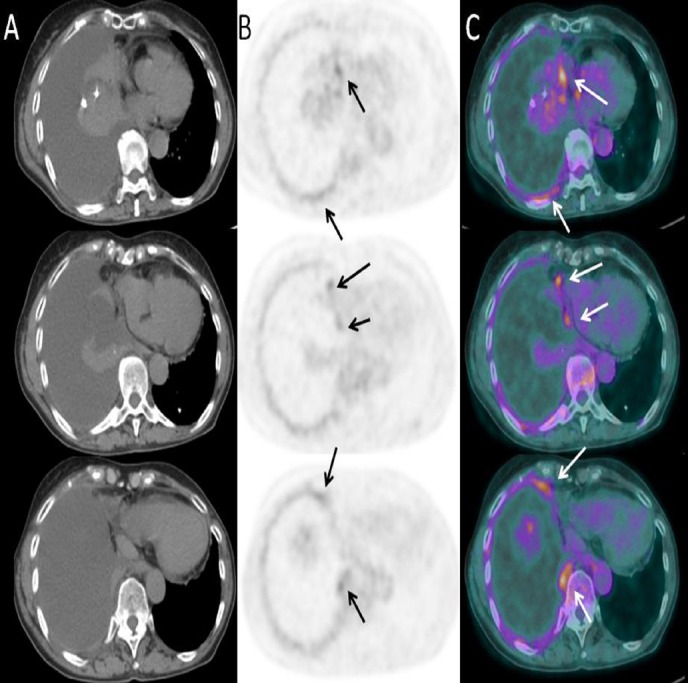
Three consecutive axial CT (A), PET (B) and fused PET/CT (C) images showing moderate FDG uptake in nodular pleural thickening of right lung (SUV_max_ 4.6) with non-FDG-avid pleural effusion
